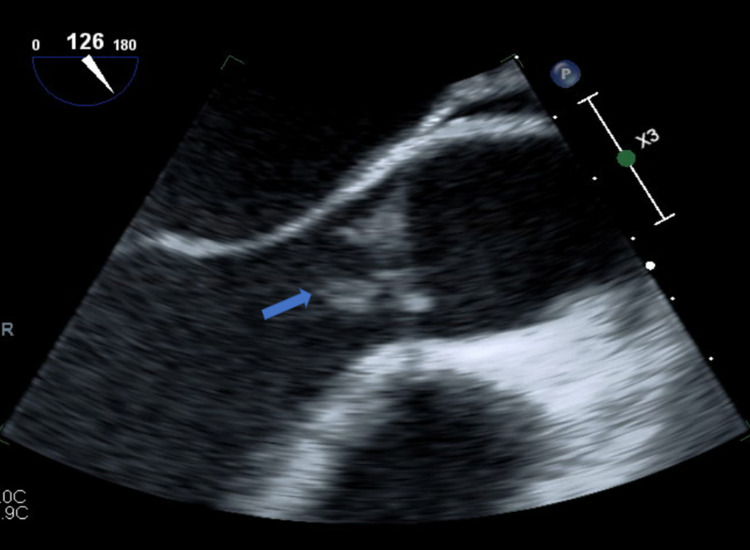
TEE showing two separate mobile vegetations (blue arrow). TEE, transesophageal echocardiography
